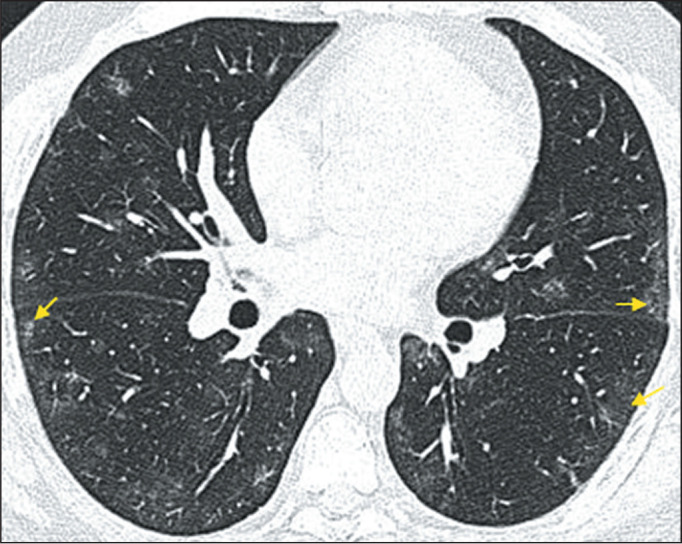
CT scan of a 47-year-old male patient, showing discrete ground-glass
